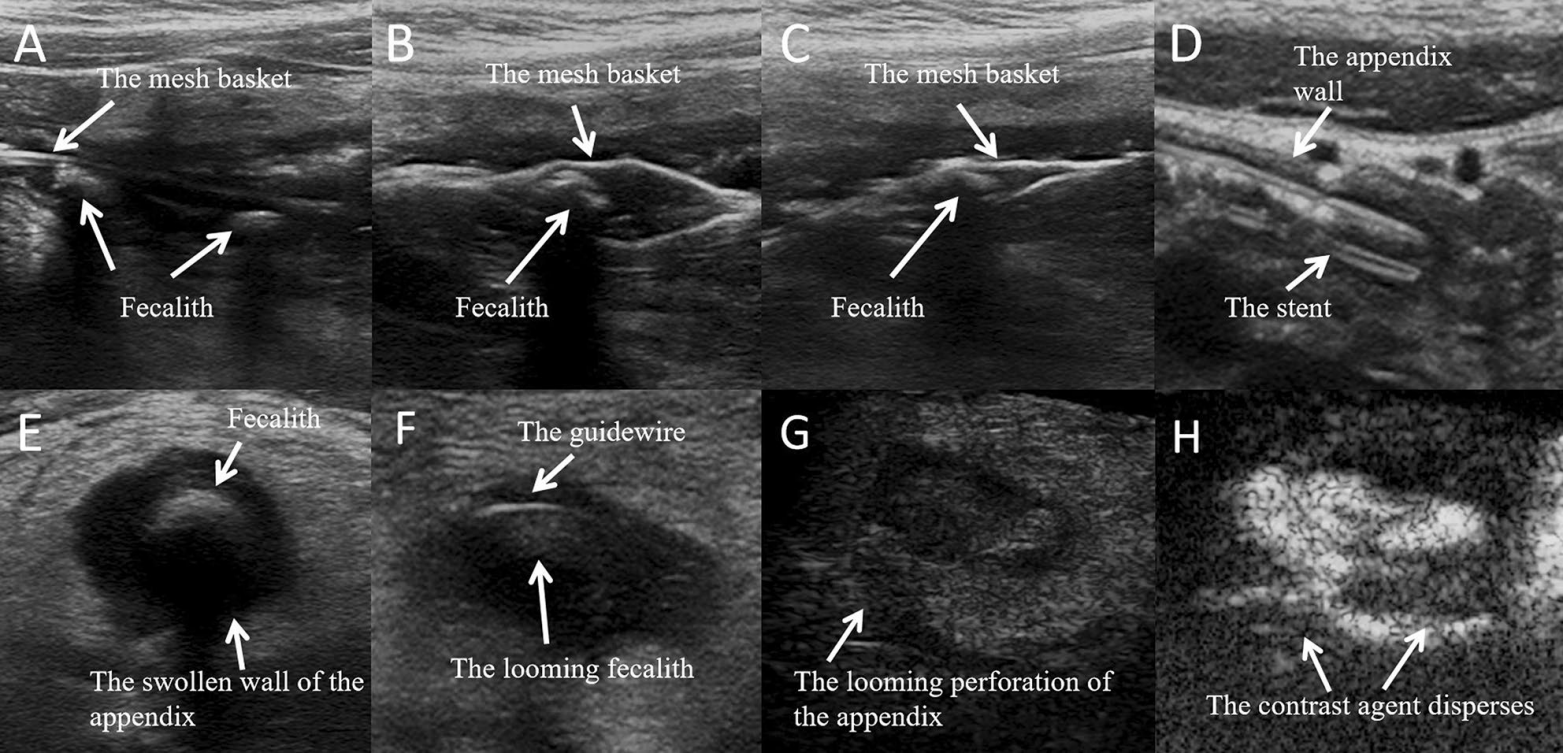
HFUS combined with intraoperative contrast-enhanced ultrasound imaging guided removal of fecalith. (A) The mesh basket slowly reached the fecalith (the diameter < 0.8 cm). (B) The mesh basket slowly opened. (C) The mesh basket rotated and tightened the fecalith. (D) The stent was placed after the fecalith was successfully removed. (E) There was a fecalith (the diameter ≥ 0.8 cm) with hard texture in the appendix cavity and the wall of appendix was significantly swollen. (F) The guidewire reached the fecalith. (G) Suspicious perforation of appendix wall during fecalith removal. (H) The intraoperative contrast-enhanced ultrasound imaging in the appendix cavity founded that the contrast agents dispersed around the appendix and determined that the appendix wall was perforated.
